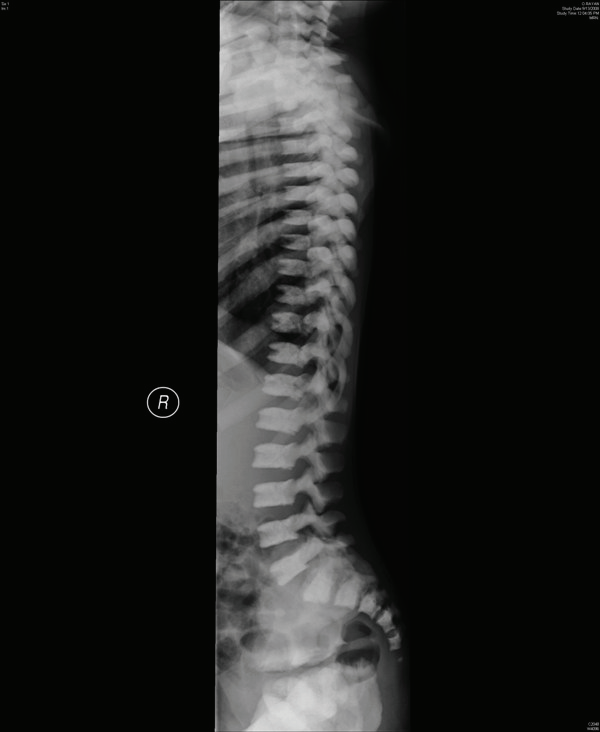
Plain radiograph of spine showing the hyperstotic vertebral endplates.
